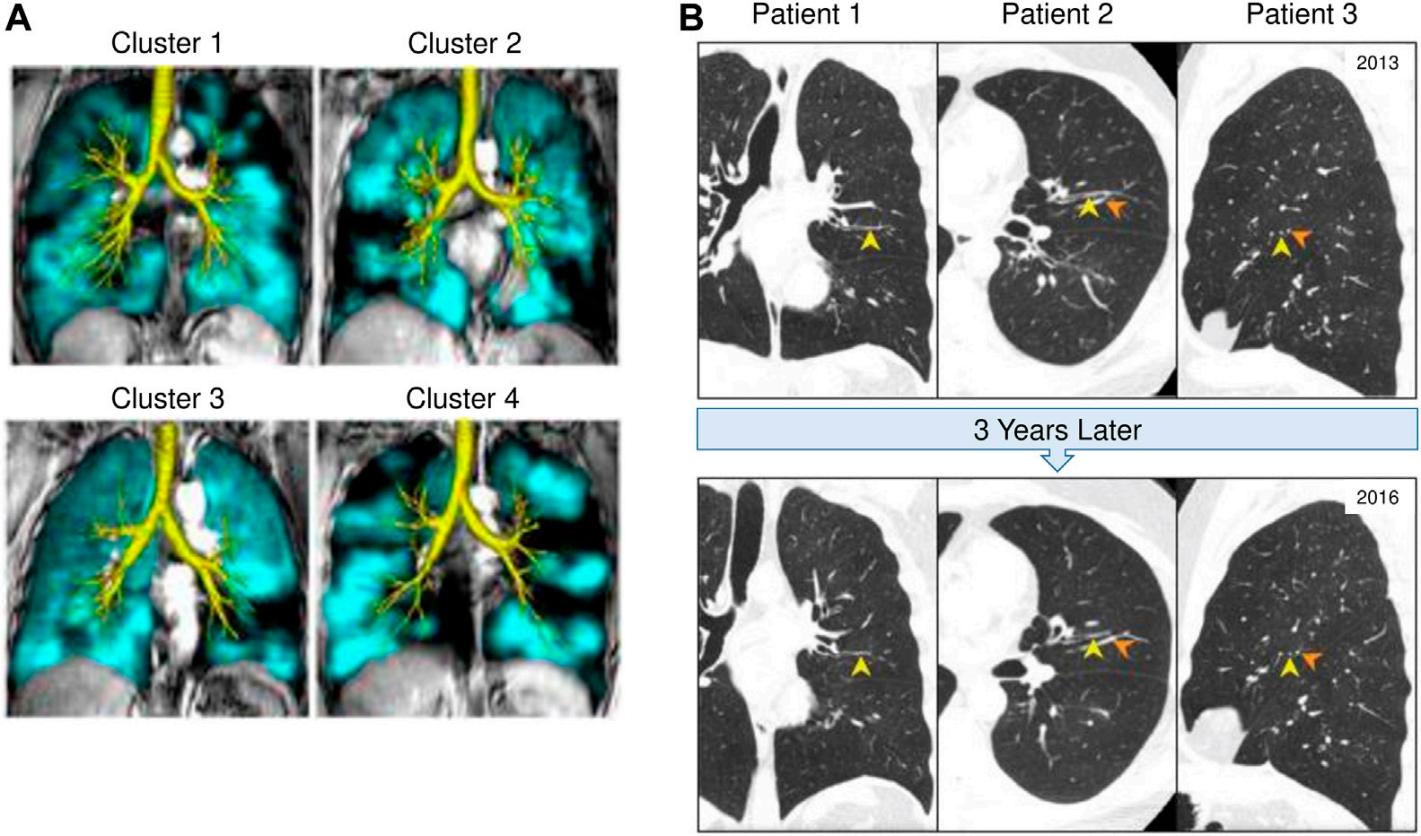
In vivo* imaging of mucus plugging and ventilation defects in CT and MRI. (A) Lung computed tomography (CT) scans from three different asthma patients (A1, A2, and A3), which demonstrate the persistence of mucus plugs (Yellow arrowheads) in the same airway at baseline (2013) and 3 years later (2016). Orange arrowheads indicate blood vessels. This figure was adapted with permission from Figure 1 by Tang M et al. published in the American Journal of Respiratory and Critical Care Medicine 2022. (B)
